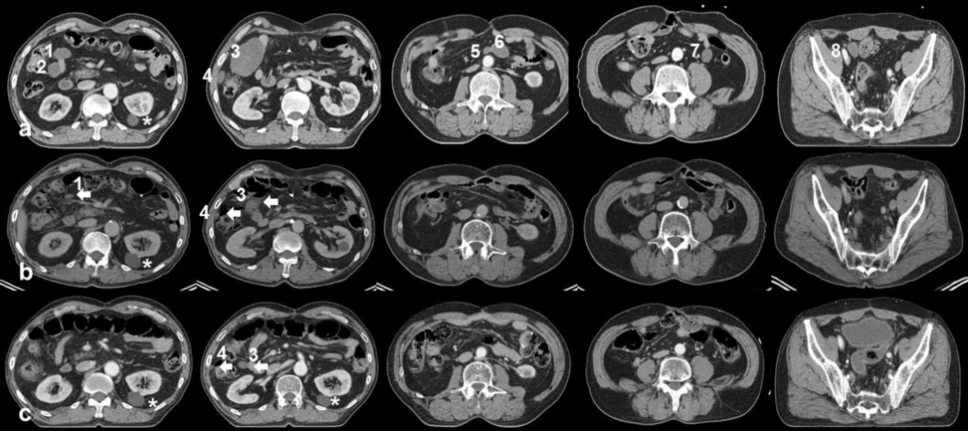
Transverse images of a contrast-enhanced abdominal computed tomography scan showing a regression of the peritoneal metastases. (a) An abdominal computed tomography scan from September 2013, showed multifocal metastatic peritoneal nodules (numbered from ‘1’ to ‘8’) before intraperitoneal radiotherapy. (b) Surveillance by computed tomography scan, obtained in December 2013 after completion of radiotherapy, showed a complete response of five nodules (1, 2, 5, 6, 7, and 8) and a partial response of three nodules (1, 3, and 4). (c) A computed tomography scan, obtained in March 2014 after completion of radiotherapy, showed that most of the metastatic peritoneal lesions had disappeared, except two small nodules that had decreased in size by about 0.8–1 cm (3 and 4). The asterisk (*) indicates a simple benign cyst.
